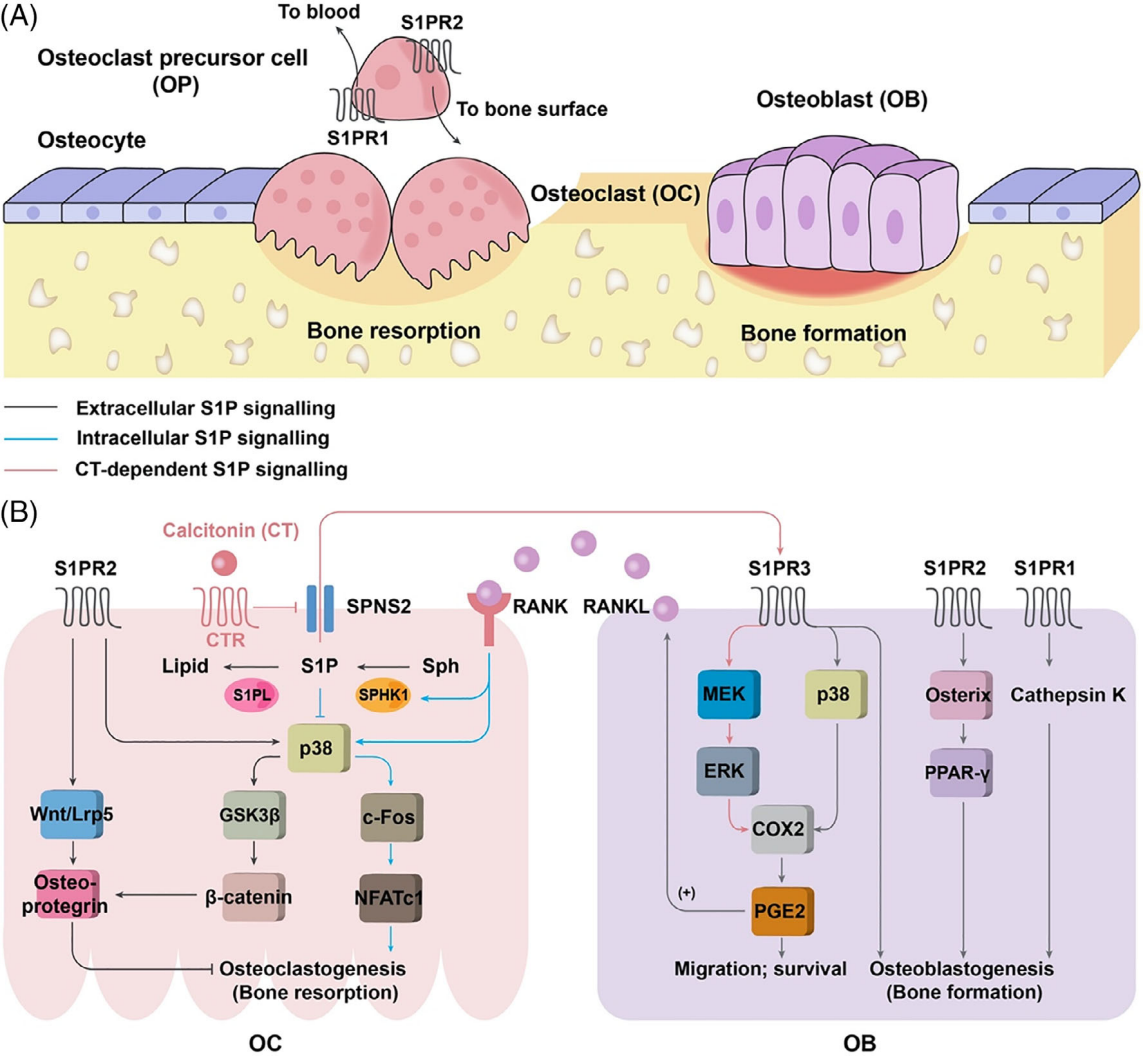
Sphingosine 1‐phosphate (S1P) signalling in bone morphogenesis. (A) Bone morphogenesis involves a highly dynamic regulation of bone resorption and formation by osteoclasts (OCs) and osteoblasts (OBs). Extracellular S1P secreted from osteoclast precursor cells promotes circulation of OCs between blood and bone through S1P receptor 1 (S1PR1)‐directed OCs migrating away from bone and S1PR2‐mediated OCs migrating towards bone. (B) Osteoclastogenesis is inhibited by both extracellular S1P signalling [through S1PR2/wingless‐related integration site (Wnt)/lipoprotein receptor‐related protein 5 (Lrp5) and S1PR2/glycogen synthase kinase 3β (GSK3β)/β‐catenin‐induced osteoprotegrin upregulation] and intracellular S1P signalling [through negative regulation of the p38/FBJ osteosarcoma oncogene (c‐Fos)/nuclear factor of activated T cells 1 (NFATc1) pathway]. Extracellular S1P secreted from OCs [negatively regulated by calcitonin (CT) and calcitonin receptor (CTR)] promotes the migration and survival of OBs by cyclooxygenase‐2 (COX2) and prostaglandin E2 (PGE2)‐induced receptor activator of NF‐κB ligand (RANKL) upregulation through modulating activities of p38 and extracellular signal‐regulated kinase (ERK) in OBs. RANKL secreted from OBs binds with RANK in OCs and activates SPHK1, which, together with S1P lyase (S1PL), in turn regulates S1P metabolism. In addition, S1PR1‐ and S1PR2‐mediated S1P signalling stimulates osteoblastogenesis by regulating osterix/peroxisome proliferator‐activated receptor γ (PPARγ) and cathepsin K, respectively. MEK, mitogen‐activated protein kinase kinase; SPNS2, S1P transporter spinster homolog.
